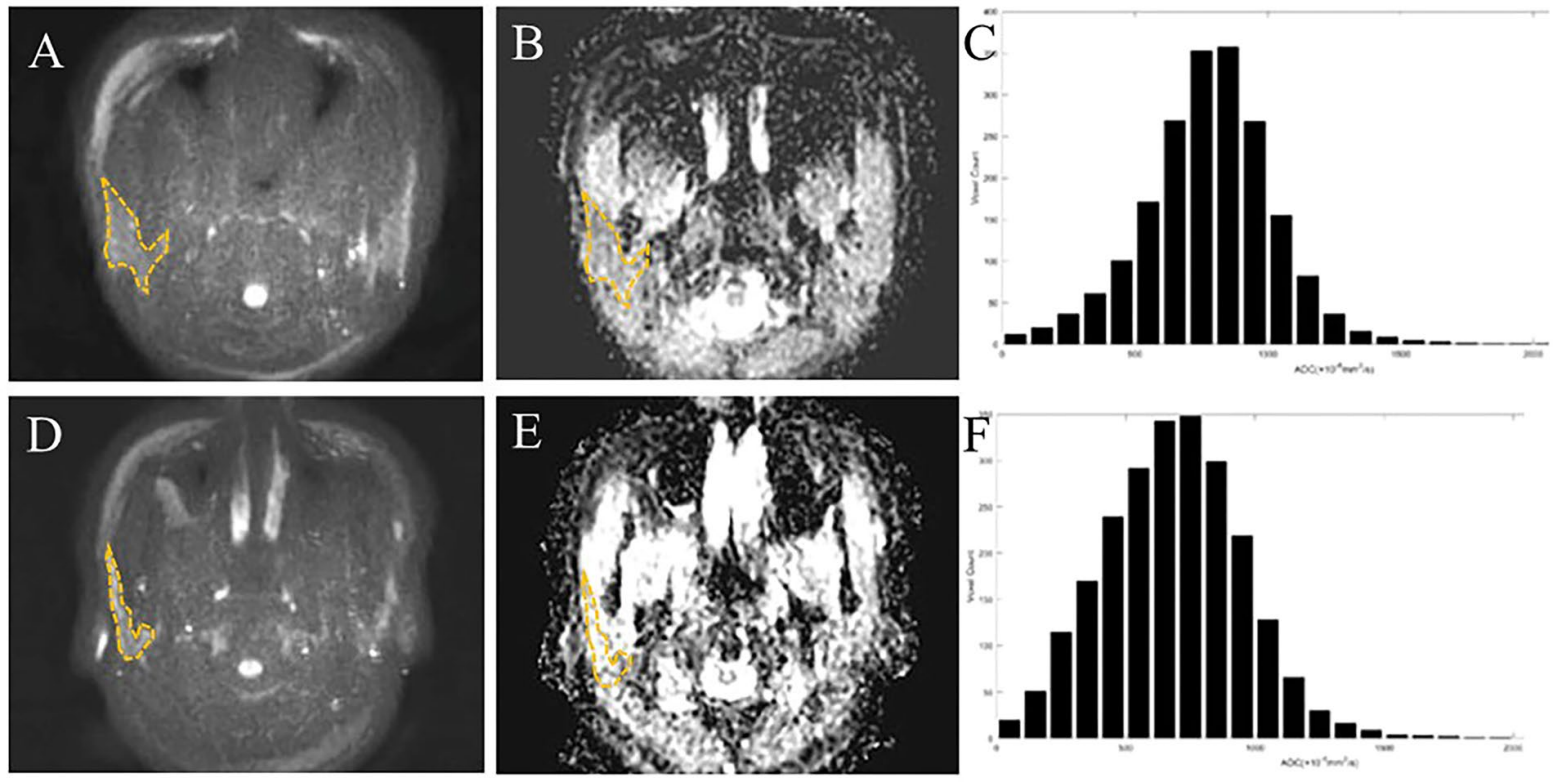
(A–C) Axial diffusion-weighted imaging (DWI) scan (*b* = 1000 s/mm^2^), corresponding to the apparent diffusion coefficient (ADC) map, and histogram of the bilateral parotid glands of an 18-year-old woman with low-activity primary Sjögren’s syndrome (pSS). Her European League Against Rheumatism (EULAR) pSS disease activity index (ESSDAI) score was 4 (haematological score 2+ serum biomarker score 2). MRI morphology, ANA, anti-SSA, X-ray sialography, and lip biopsy showed positive findings, whereas her anti-SSB and ocular tests were negative. The parotid ADC, ADC_mean_, skewness, kurtosis, and entropy values were 959.1 × 10^−6^ mm^2^/s, 1006.2 × 10^−6^ mm^2^/s, −0.177, 4.746, and 5.690, respectively. (D–F) Axial DWI scan (*b* = 1000 s/mm^2^), corresponding to the ADC map, and histogram of the bilateral parotid glands in a 66-year-old woman with moderate-activity pSS. Her ESSDAI score was 6 (haematological test, serum biological marker score 2 and constitutional symptoms, articular score 1). MRI morphology, ANA, anti-SSA, anti-SSB, and X-ray sialography showed positive findings, whereas the ocular tests and lip biopsy were negative. The ADC, ADC_mean_, skewness, kurtosis, and entropy values were 943.0 × 10^−6^ mm^2^/s, 1015.6 × 10^−6^ mm^2^/s, 0.359, 5.254, and 6.181, respectively. Note the dashed lines covering the edge of the right parotid glands.
